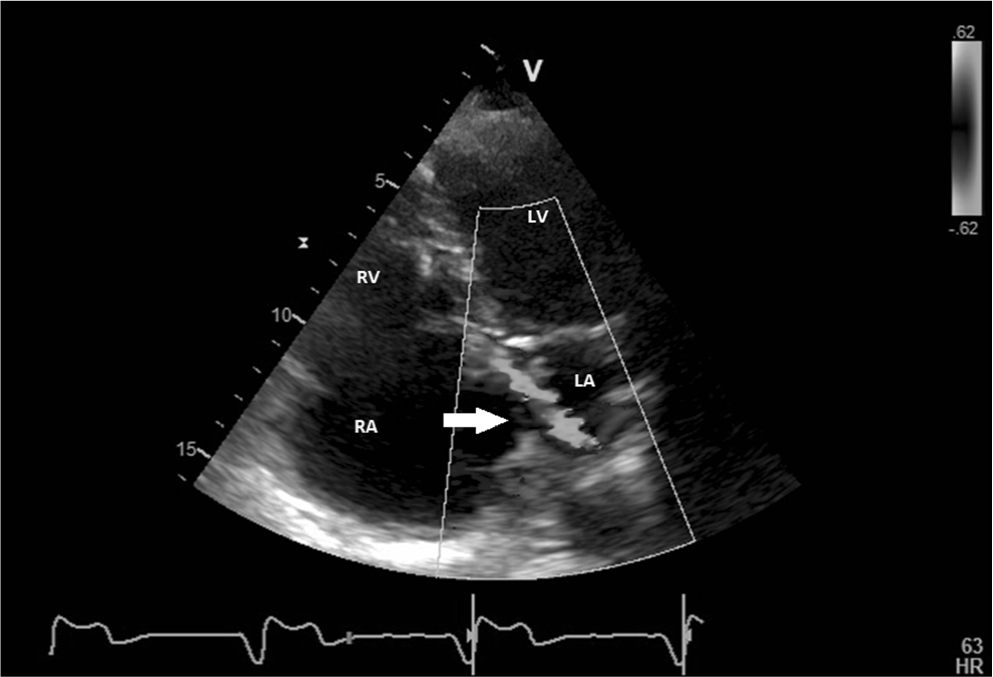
Transthoracic echocardiography, four-chamber view of patient B showing an abnormal flow signal from the roof of the left atrium to the interatrial septum on colour Doppler (*arrow*). *RA* right atrium, *RV* right ventricle, *LA* left atrium and *LV* left ventricle
